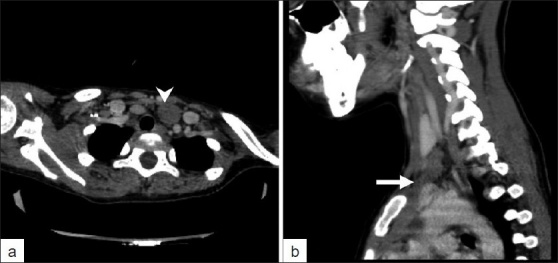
Axial and sagittal contrast enhanced CT images
